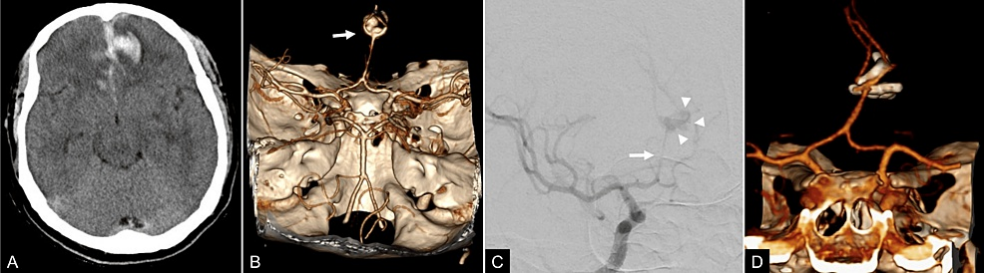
Pre- and postoperative radiological studies of case 2. (A) Computed tomography (CT) sagittal section showing a hyperdense lesion in the anterior interhemispheric fissure (AIHF) associated with subarachnoid hemorrhage. (B) Preoperative CTA with 3D reconstruction and (C) right carotid angiogram scans reveal a single A2 trunk and a multilobulated aneurysm (arrowheads) associated with important vasospasm (arrow). (D) Postoperative CTA with 3D reconstruction demonstrating the disposition of clips applied and resolution of the aneurysm.
